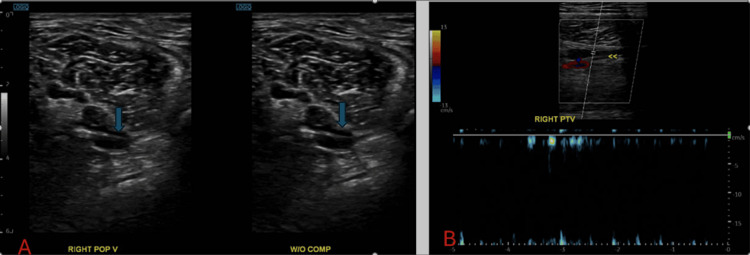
A) Doppler US of lower extremities veins shows non-compressible POP vein (arrows); B) US of right PV. The vein here is non-compressible (from last admission in 2024) US: Ultrasound; POP: Popliteal; PTV: Posterior tibial vein
